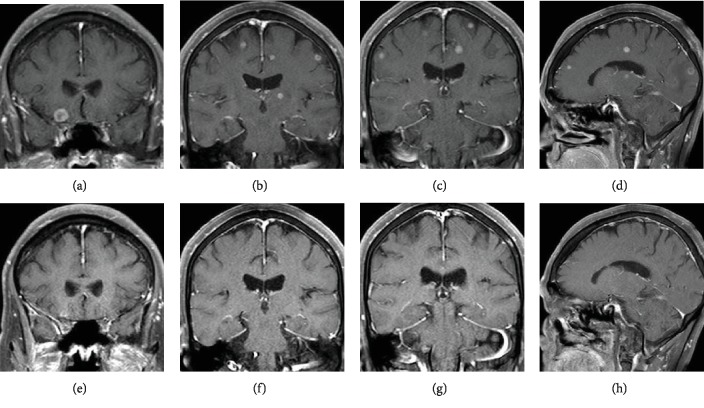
Multiple asymptomatic brain metastatic lesions (more than 20 in total) with a maximum diameter of 12 mm were detected by gadolinium-enhanced MRI at the first diagnosis (a–d). After 5 weeks, the multiple brain metastases had disappeared completely on contrast-enhanced brain MRI (e–h). Complete CNS response was confirmed.
